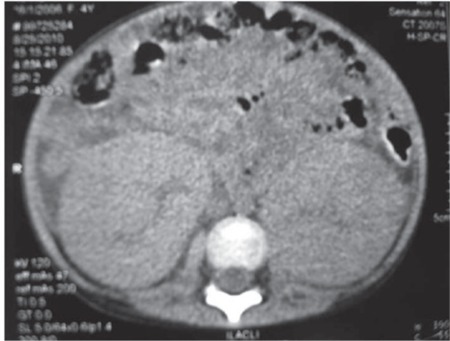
Non-contrast-enhanced axial abdominal CT scan. Significant bilateral kidney enlargement and intraabdominal free fluid are seen.
